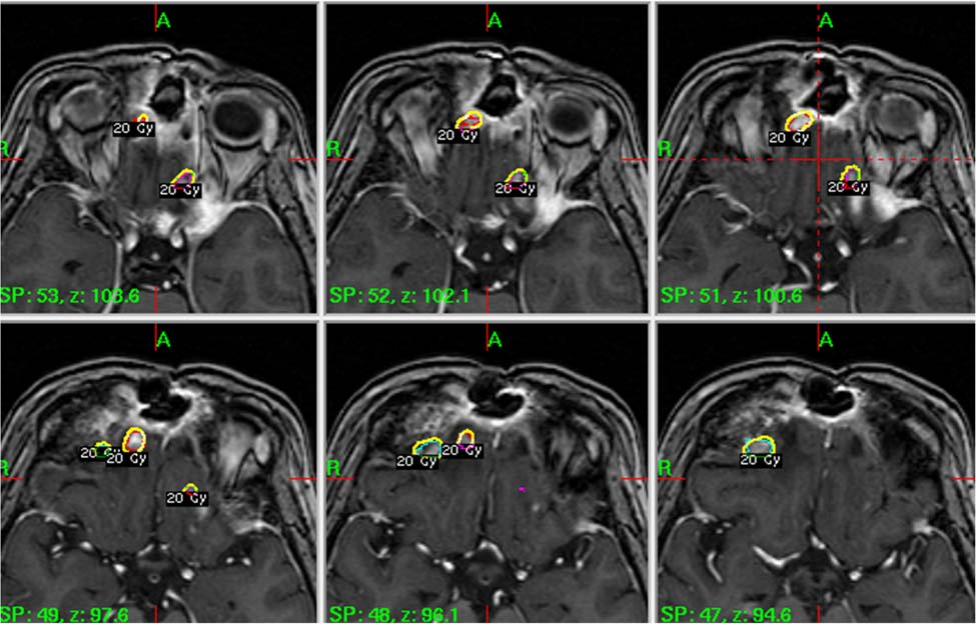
Axial T1-weighted, contrast-enhanced magnetic resonance images one year after the first treatment, showing new, small tumour foci in the left frontal lobe and above the right orbital apex. These were treated with a new session of radiosurgery. Treatment isodoses are shown as outlined in the Gamma Knife® planning software.
